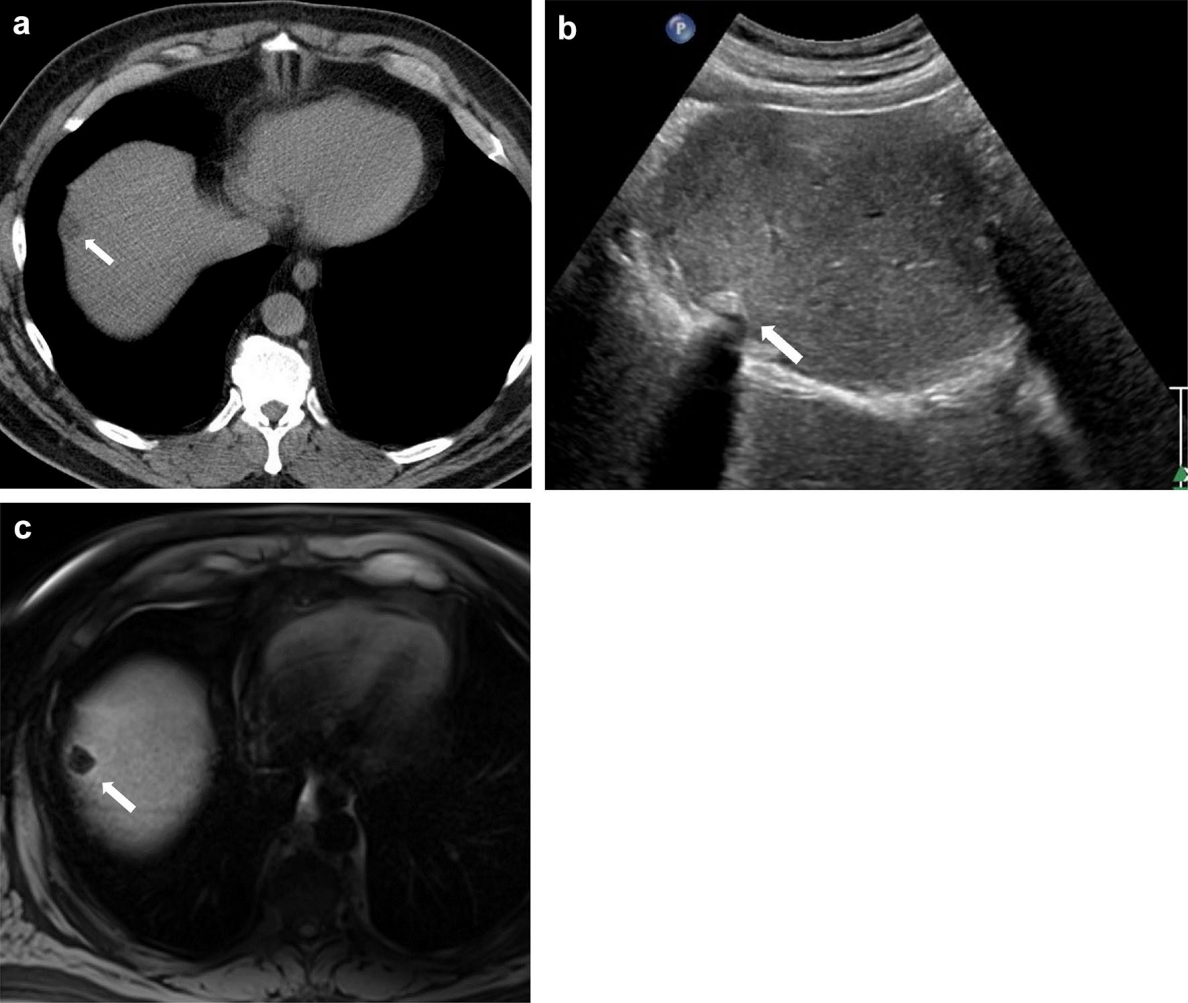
A 51-year-old male presented with dropped gallstones. He presented with intractable hiccups a few months post-cholecystectomy. A chest CT was performed for evaluation, which was unremarkable. However, an indeterminate lesion was seen in the right hepatic dome. a Axial noncontrast CT image demonstrating a hypodense lesion in the right hepatic dome (arrow). Ultrasound of the right upper quadrant was performed to further characterize this lesion. b An ultrasound image of the area of interest demonstrates a hyperechoic focus with posterior acoustic shadowing in the right subdiaphragmatic region indenting the right lobe of the liver, consistent with a dropped gallstone (arrow). c) T1-weighted MR image also demonstrates the dropped gallstone as a signal void (arrow). Multiple gallstones were seen in the subhepatic region and in the gallbladder fossa (not shown)
